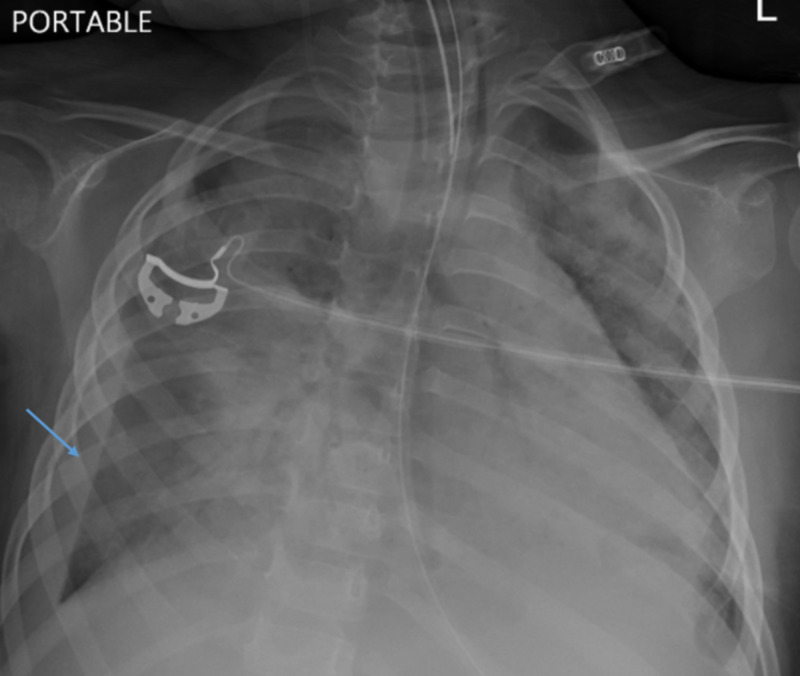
Chest X-ray demonstrating extensive bilateral lung infiltrates and a right pleural effusion.
